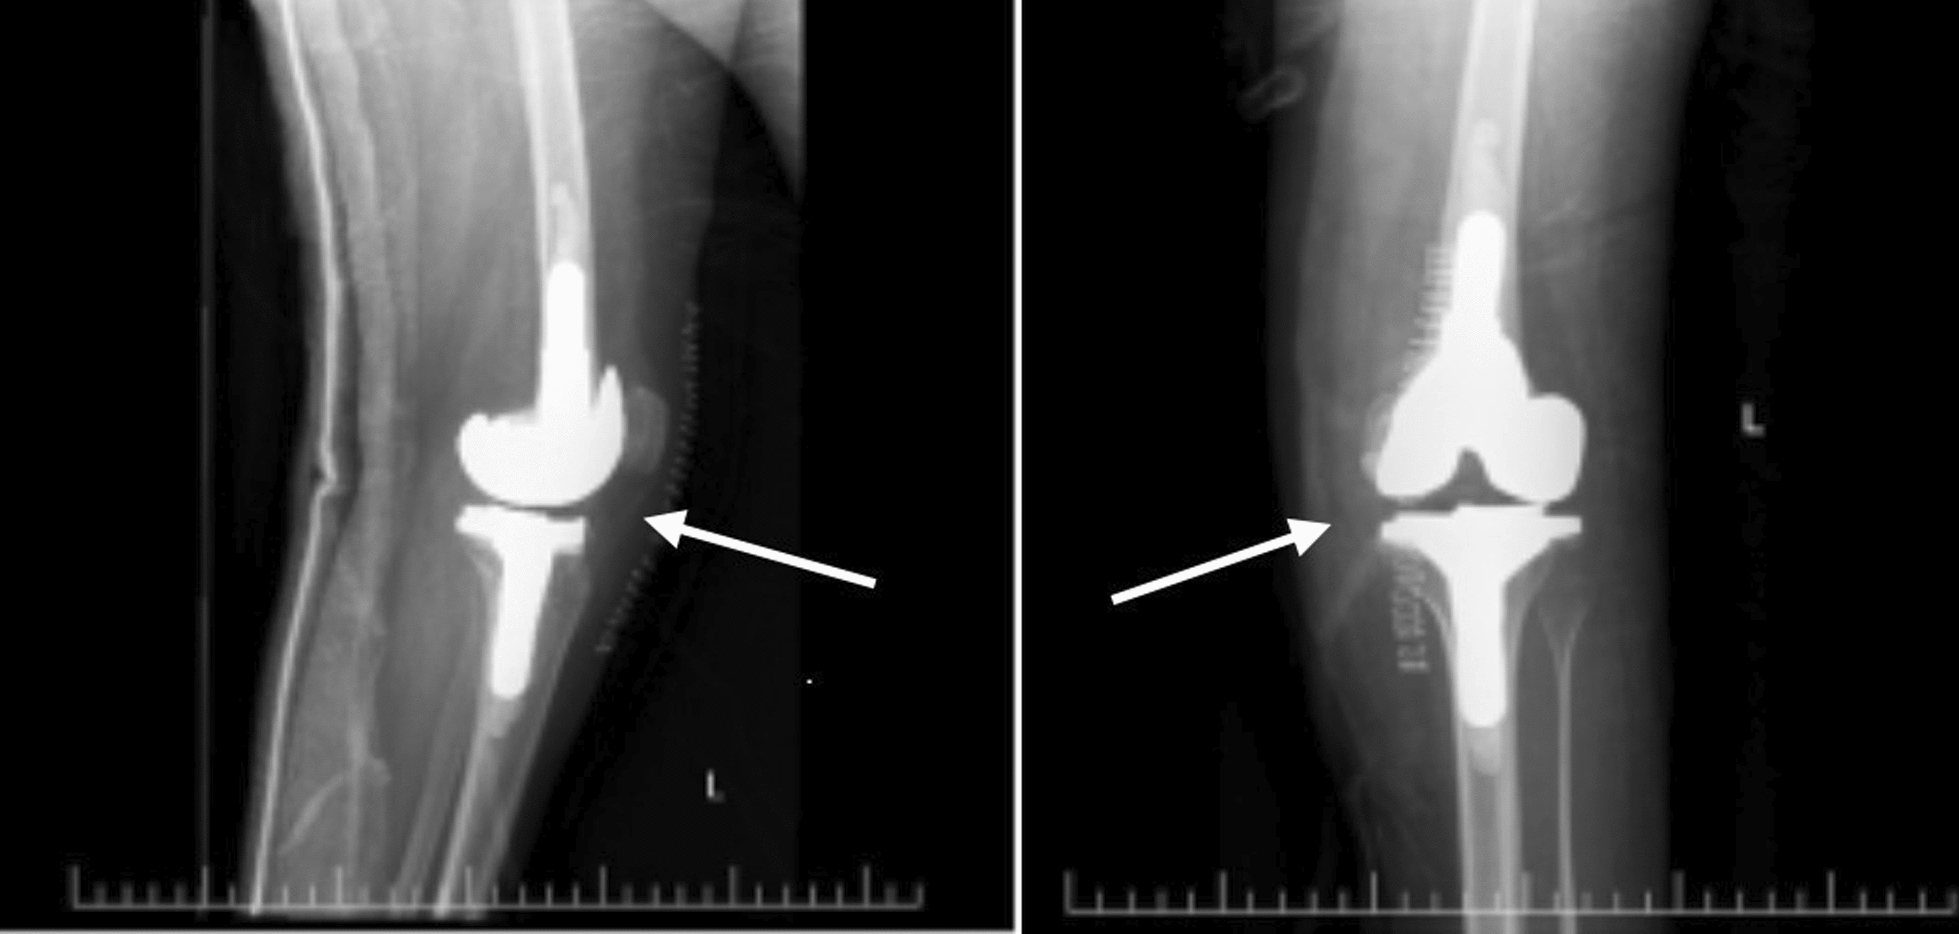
X-ray of left knee showed the implant well positioned and with good alignment
